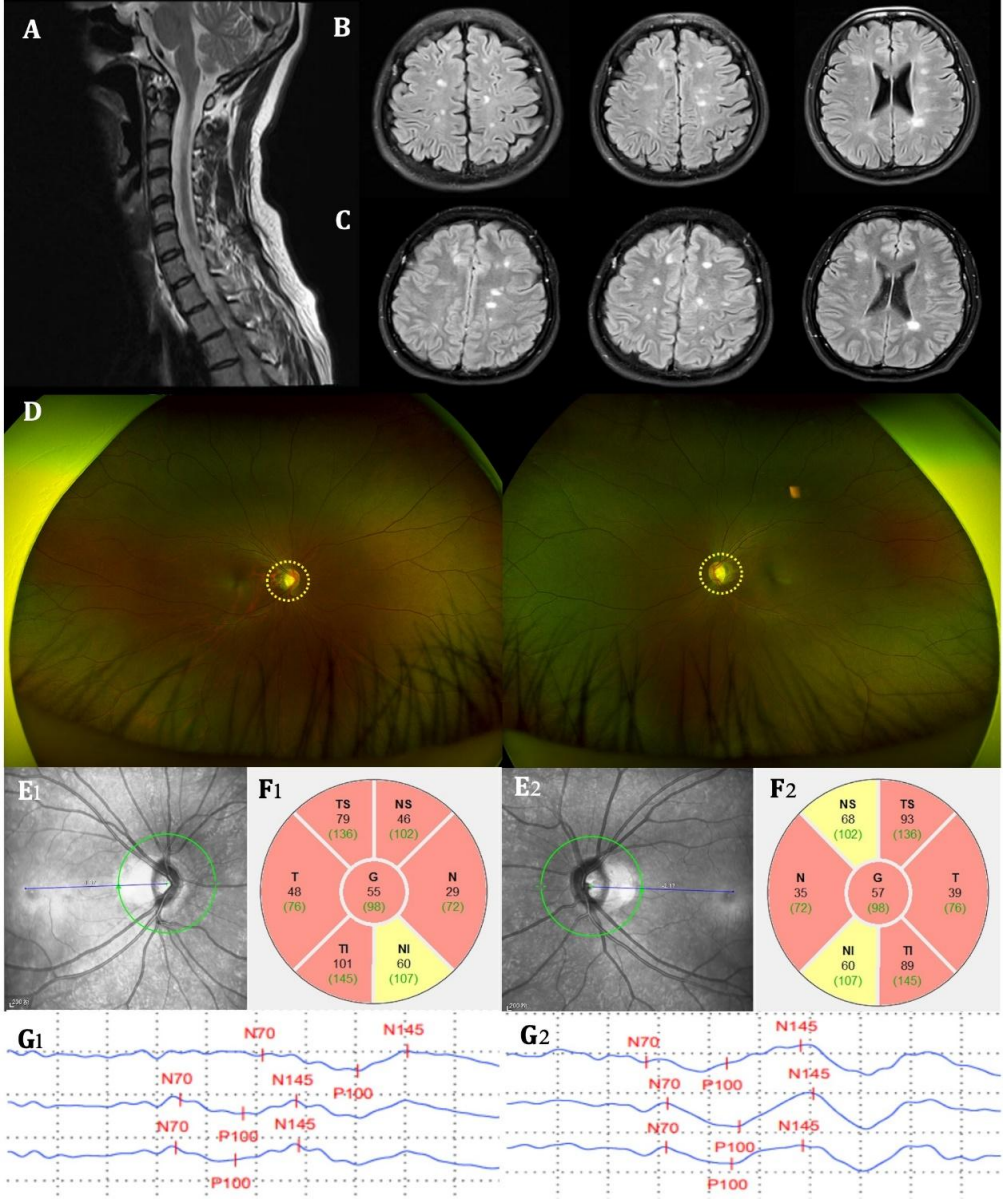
An example of OCT findings in a RRMS patient. A 33-year-old female was admitted to the hospital due to “recurrent limb weakness and numbness for 11 years, worsened for 2 days”. Previously diagnosed with RRMS in an external hospital. Baseline (September 27^th^, 2022) cervical (T2 sequence, A) and brain MRI (T2-FLAIR sequence, B) both found multiple hyperintensities. Positive oligoclonal bands were found in CSF. Four months later (December 13^th^, 2022), the follow-up brain MRI (T2-FLAIR sequence, C) showed a change in the number of hyperintensities. During this follow-up process, the patient's fundus photography revealed a pale color of the optic discs in both eyes (yellow circles, D). OCT also confirmed optic disc atrophy (green circles, E1-2) and reduced pRNFL thickness (black numbers, F1-2) in both eyes. VEP test showed prolonged latency and reduced amplitude of binocular recorded waves (G1-2). These fundus imaging findings indicate that this RRMS patient has experienced pronounced optic nerve damage as a result of recurrent episodes of disease remission. RRMS, Relapsing-remitting multiple sclerosis; MRI, magnetic resonance imaging; CSF, cerebrospinal fluid; OCT, optical coherence tomography; pRNFL, peripapillary retinal nerve fiber layer; VEP, visual evoked potential. G, general; N, nasal; NS nasal superior; NI nasal inferior; T, temporal; TS temporal superior; TI temporal inferior.
